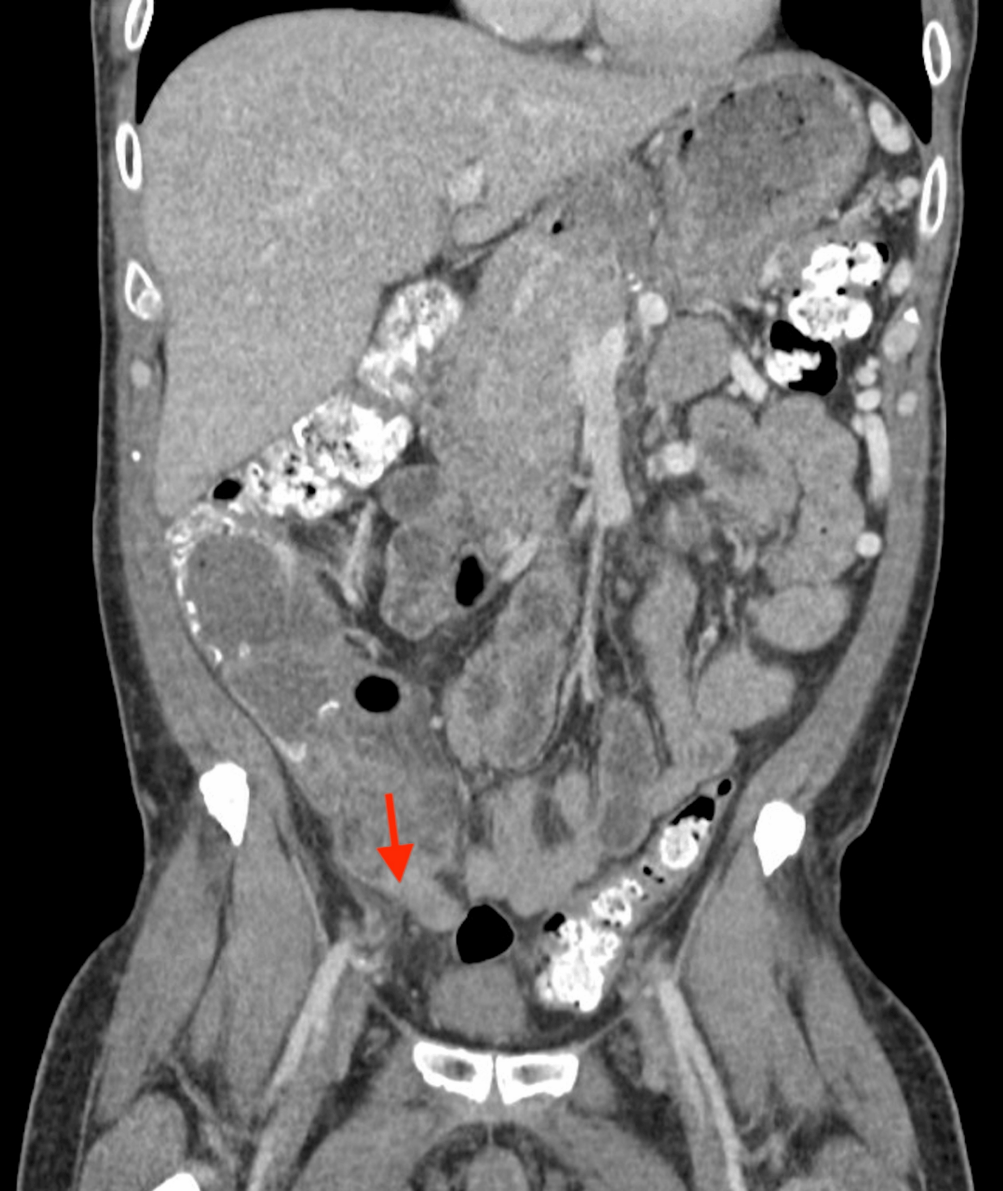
CT abdomen (coronal view) findings showing appendiceal thickening w/ surrounding inflammation in RLQ consistent with appendicitis CT: computed tomography, RLQ: right lower quadrant
